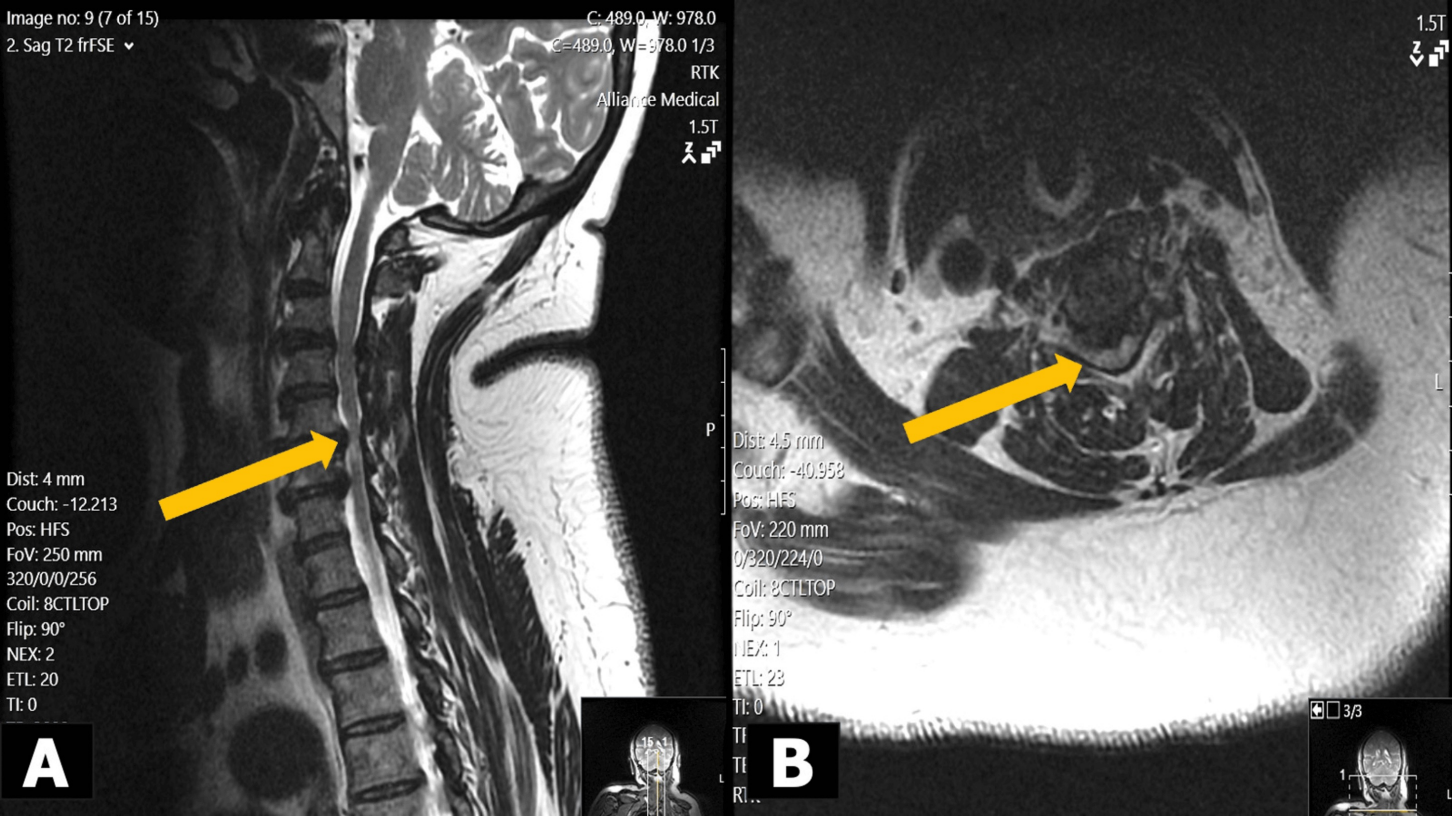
Preoperative T2-weighted MRI scans showing multilevel cervical foraminal narrowing with multilevel nerve root impingement of the right C4 and left C5 nerve roots (yellow arrows) in a) sagittal view and b) axial view.
